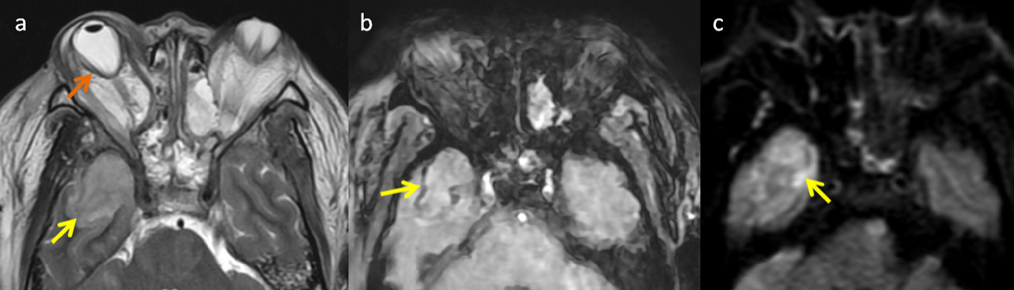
Axial MR images of mucomycosis with cerebritis. (a) Abnormal T2 hyperintense involving anterior pole of right temporal lobe (yellow arrow), orbital cellulitis with proptosis and resultant posterior globe tenting (“Guitar pick” sign) (orange arrow). (b) Rim of blooming shows in SWI images indicating fibrocollagenous capsule formation. (c) Restricted diffusivity on DWI images (arrow).
